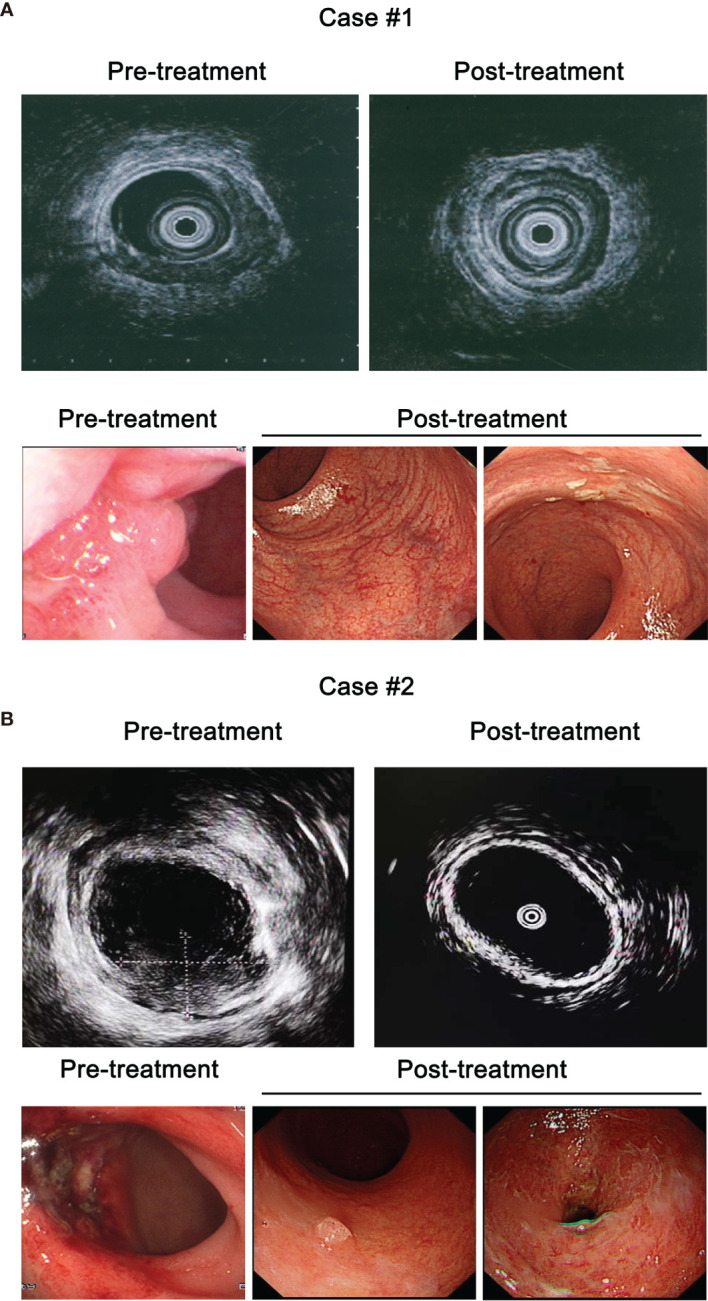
Clinical images of representative patients in the current study. (A) Pre- and post-treatment colonoscopic endoanal ultrasound scanning (EUS, upper panel) and colonoscopy (lower panel) images of case #1 with T2 tumor. (B) Pre- and post-treatment EUS (upper panel) and colonoscopy (lower panel) images of case #2 with T3 tumor.
